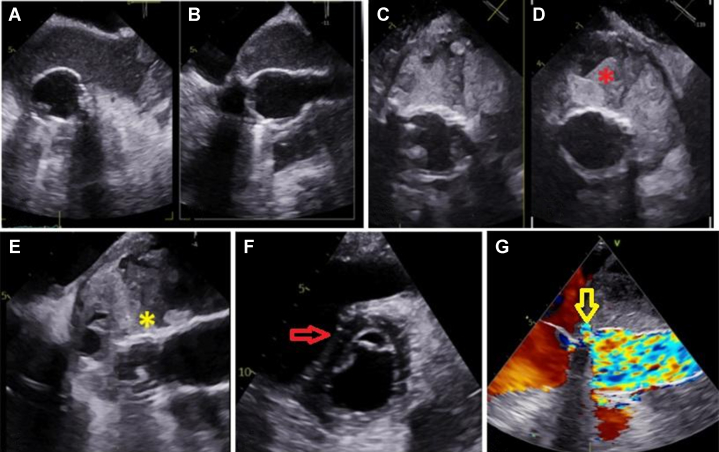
Findings on Transesophageal Echocardiography Transesophageal echocardiography demonstrating a large perigraft fluid collection surrounding the aortic conduit (A and B), with heterogeneous and thickened internal material compatible with abscess and debris (C and D). Mobile echodensity suggestive of vegetation (yellow asterisk) attached to the prosthetic aortic graft (E). (D) Red asterisk indicates the periprosthetic abscess adjacent to the aortic graft. (E) Yellow asterisk indicates the prosthetic aortic conduit and vegetation attached to the aortic graft. Marked diastolic interventricular septal flattening (red arrow) indicating elevated right-sided pressures (F). A focal jet on color Doppler (yellow arrow) demonstrates flow between the perigraft cavity and the proximal Bentall anastomosis line (G).-Blood cultures: positive for *Enterococcus faecalis* (all bottles from 4 sets of blood cultures).-Urgent CT angiography: rapid expansion of the perigraft collection with compression of adjacent structures (Figure 2).
